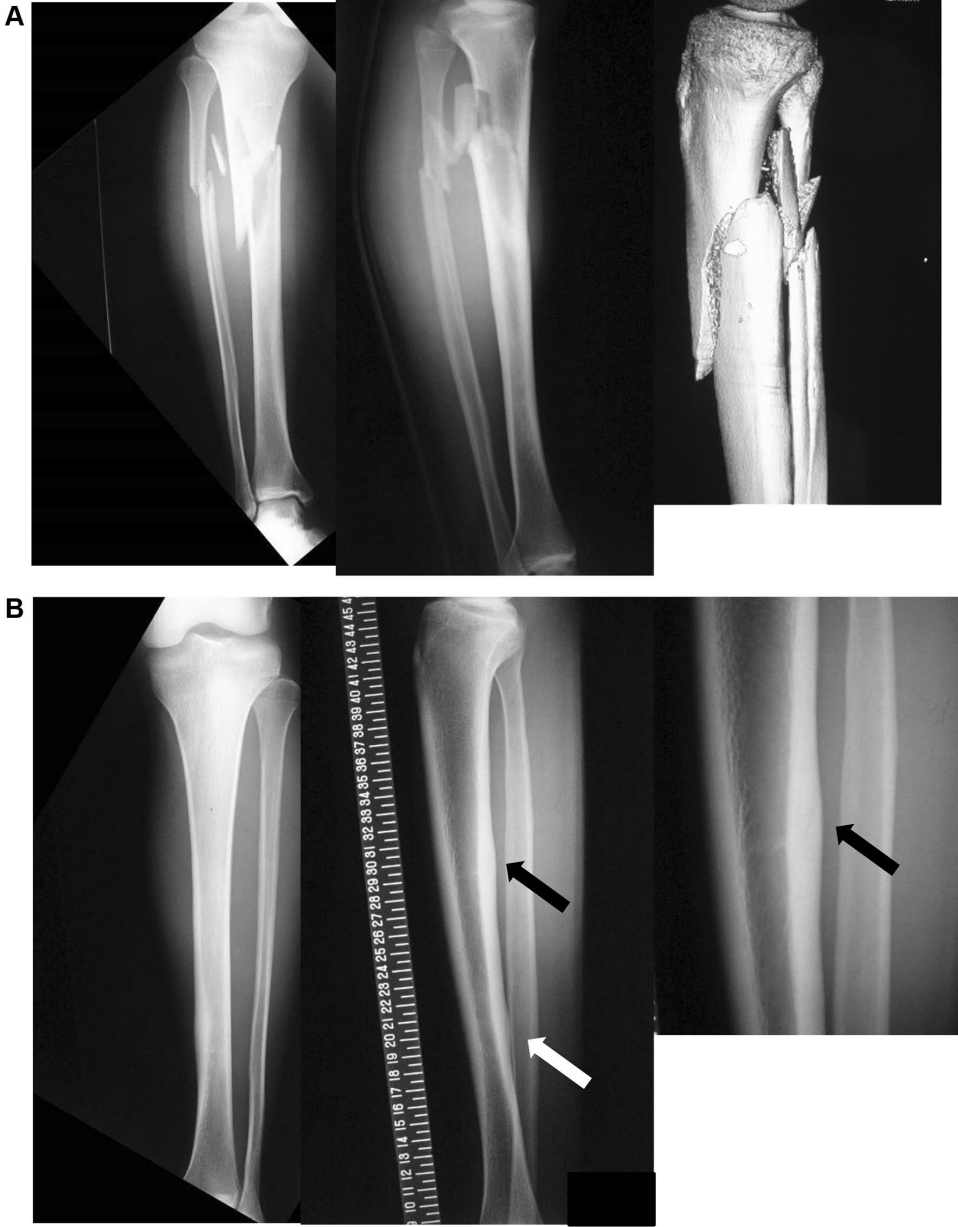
Radiographs at the emergency department with deformity of the right lower leg. Full-length tibial radiographs were requested in keeping with the clinical picture, and they confirm complete tibial and fibular fractures of the right side. A Right-side tibial radiography and 3D computed tomography; B Left-side tibial posterior stress fracture. Arrows indicate callus formation sites
